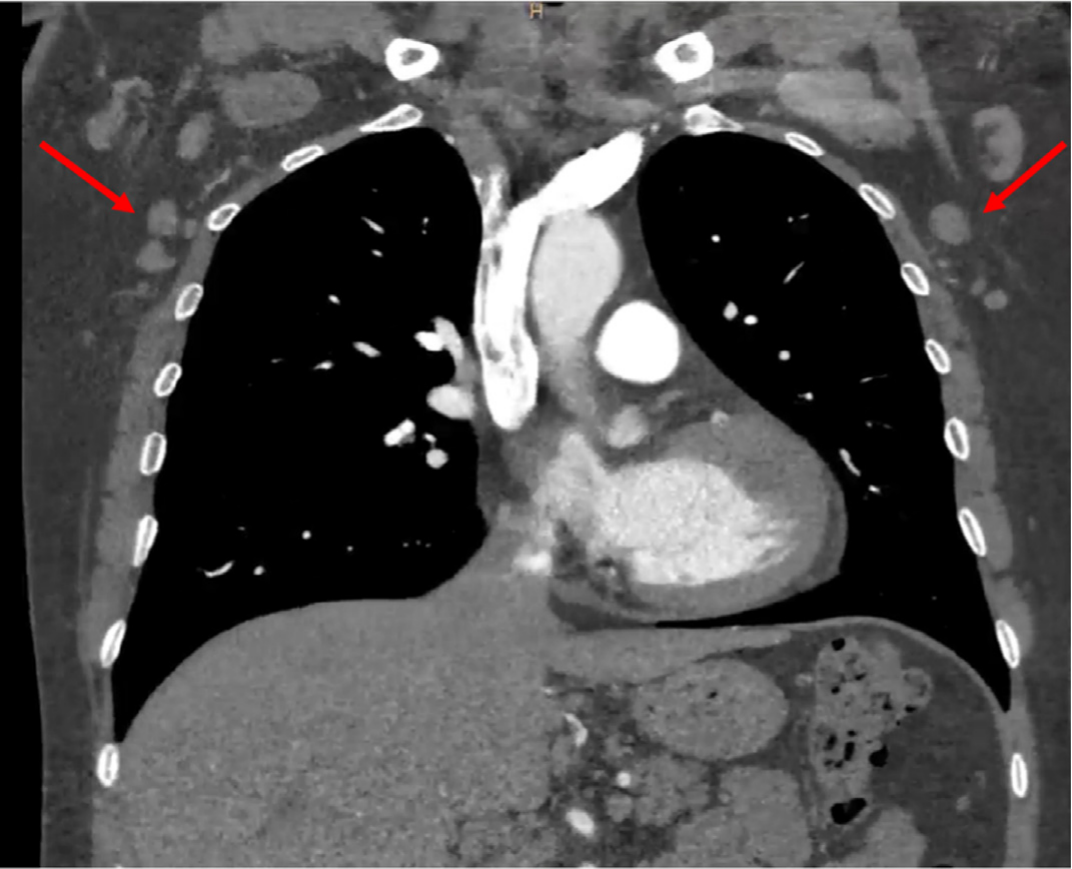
Computed Tomography Scan The image shows several small axillary and mediastinal adenopathies (arrows).
